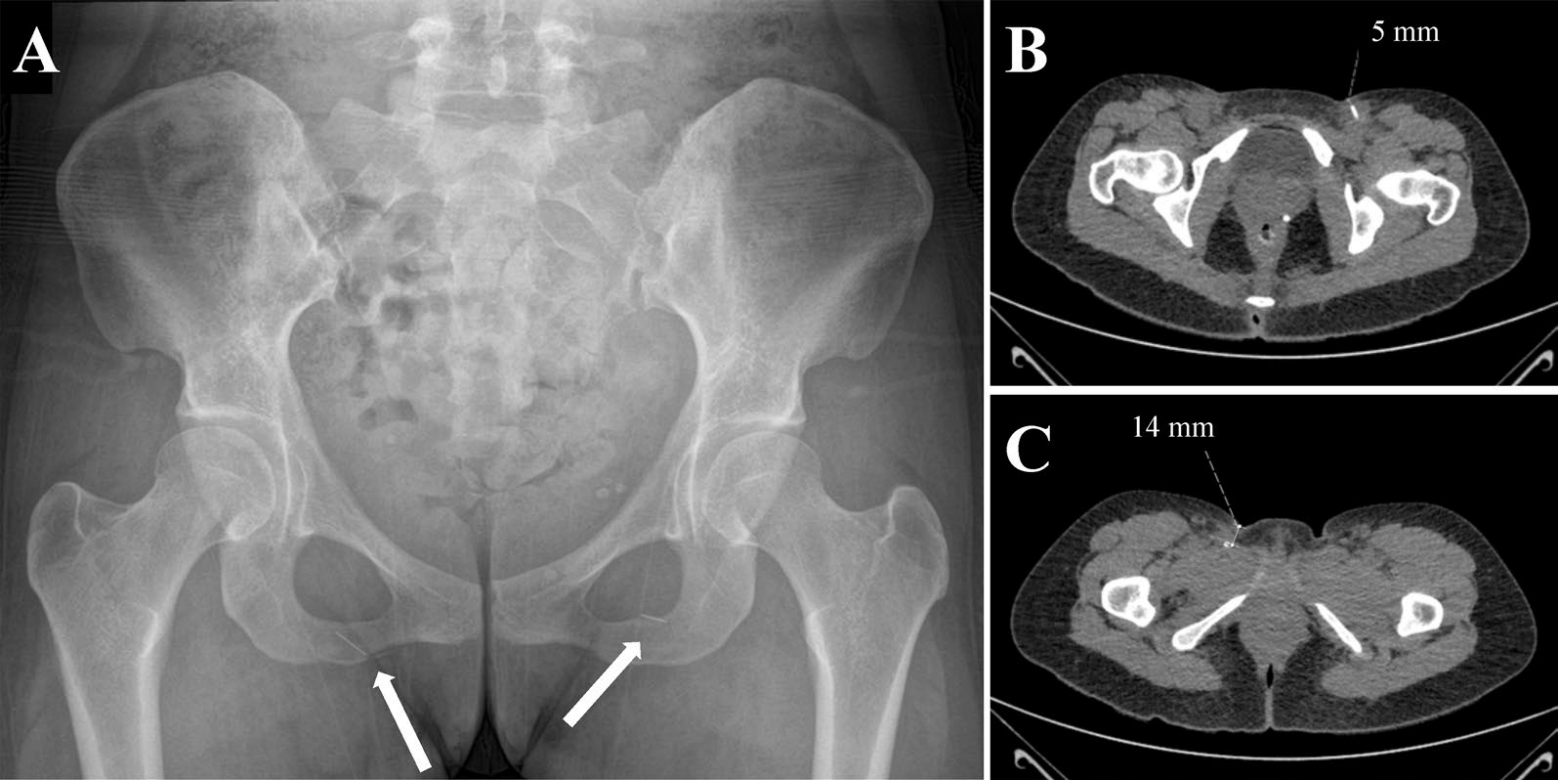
A young female patient who injects drugs with two broken needle fragments roughly 10 mm long. The fragments were visible on a plain radiograph (A). The fragments could not be visualized in the ultrasound assessment. The patient underwent subsequent computed tomography imaging to precisely locate the fragments. The fragment on the left side was close (5 mm) to the skin and adjacent to the femoral artery and veins (B). The tip of the fragment on the right side was located 14 mm from the skin; however, it did not pose a threat to either arterial or venous structures
